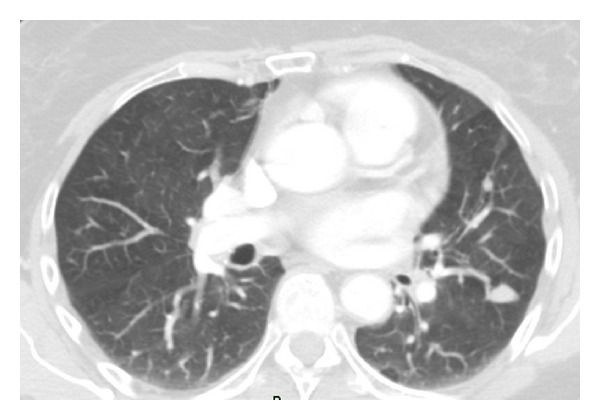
CT scan with lung metastases.
